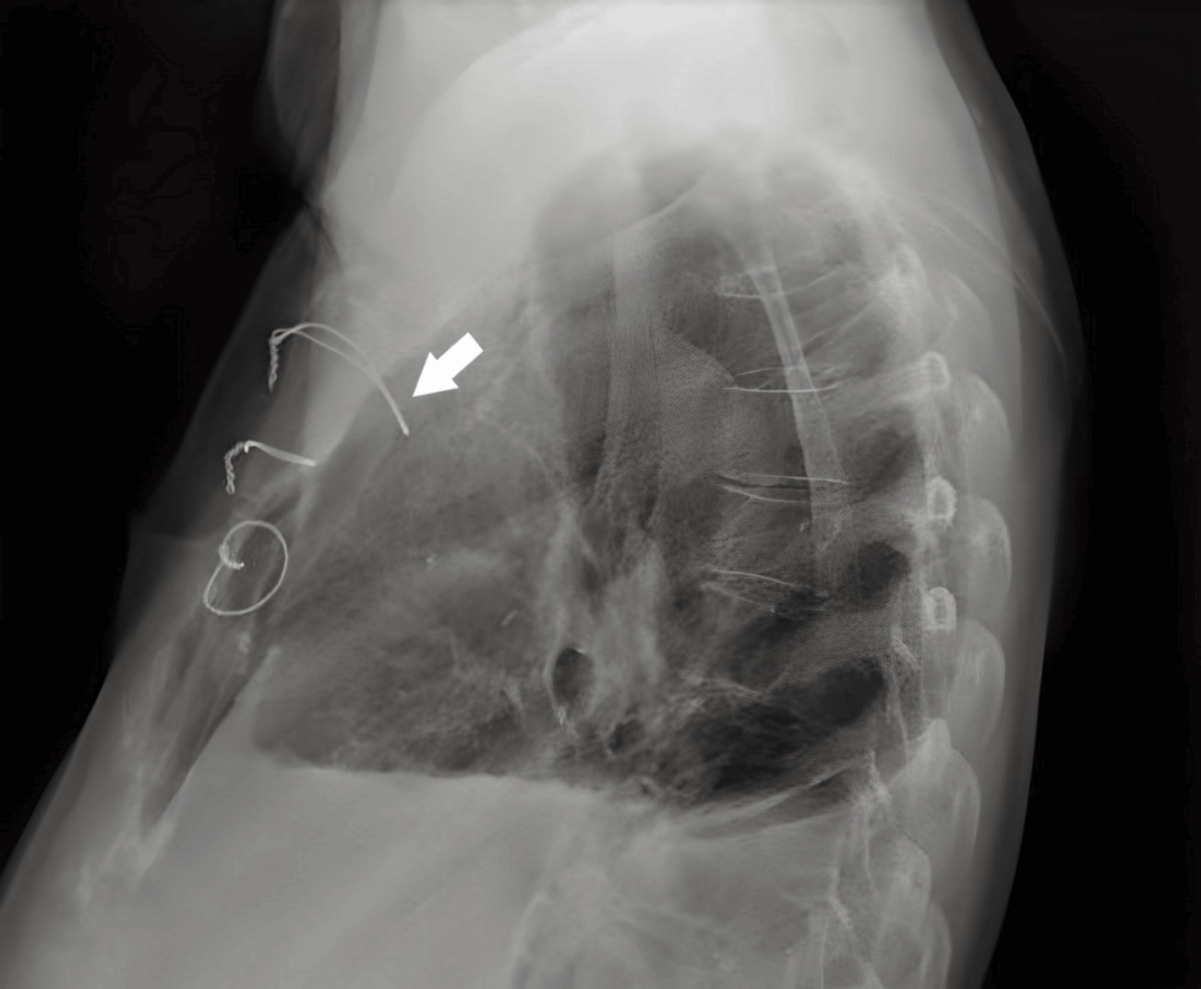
Chest X-ray showing protrusion of the loop of the first sternal wire into the anterior mediastinum
